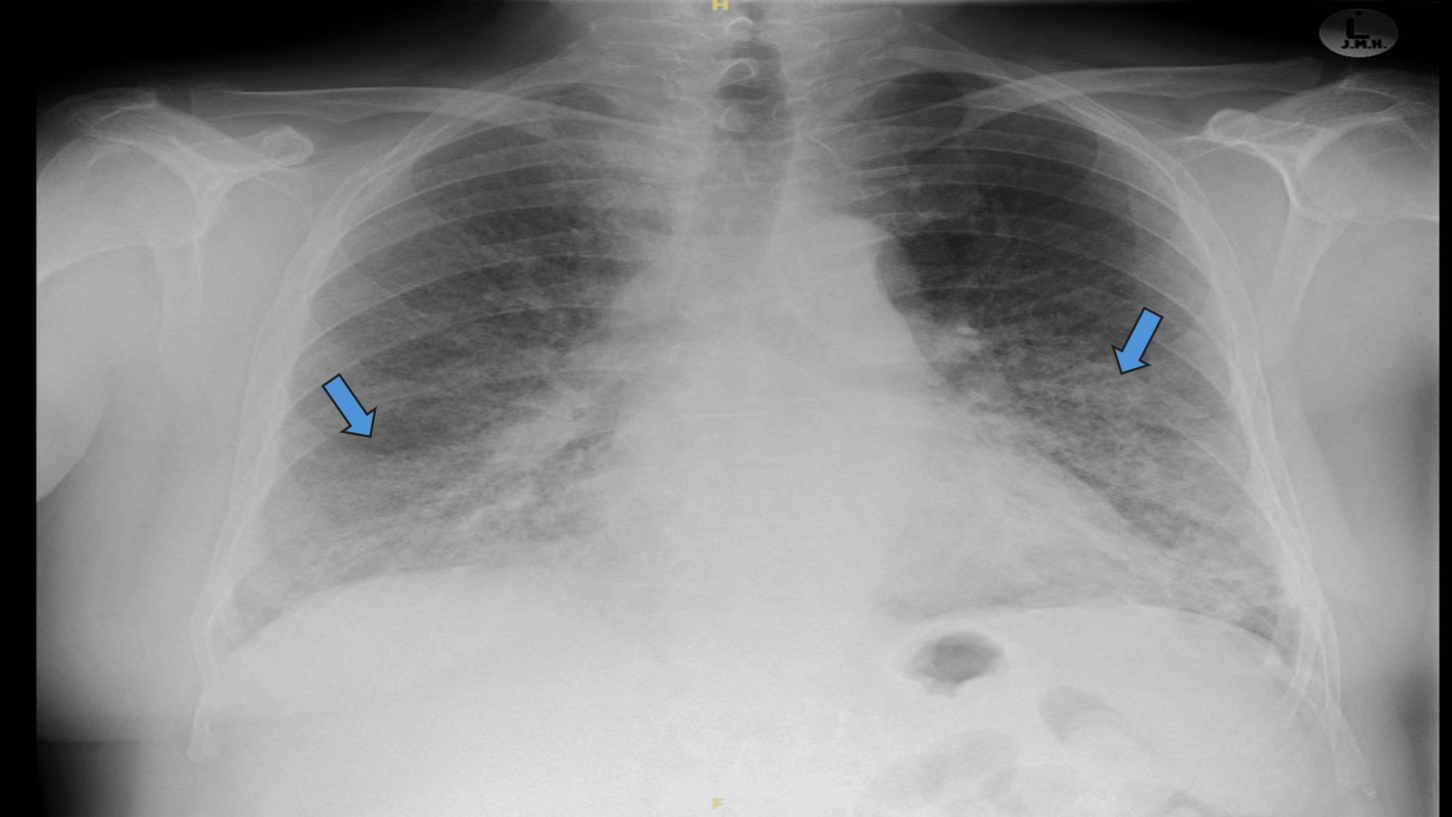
Chest X-ray from hospitalization 14 days prior to current admission Chest X-ray revealed patchy air space opacification in both the mid and lower lung zones, consistent with ongoing infections.
